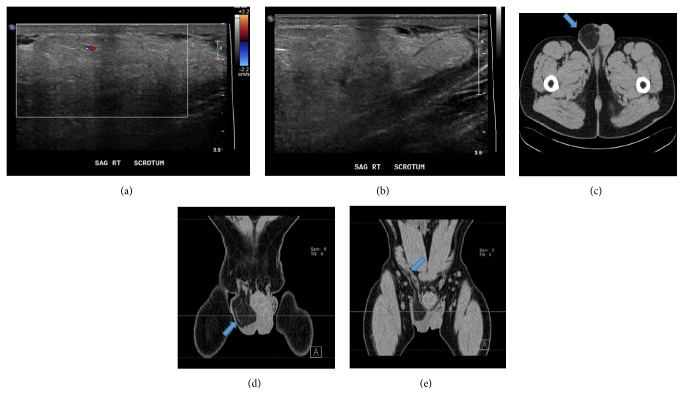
Doppler ultrasound in case 1 revealed a hyperechoic, solid, and heterogeneous lesion with minimal flow (a), extending to the scrotum (b). CT imaging in case 2 showed a low density mass with septations in the right scrotal sac ((c), axial view). The bulky mass was compressing the testicle ((d), coronal view) and showed spread into the abdominal cavity through the inguinal canal ((e), coronal view, arrow).
